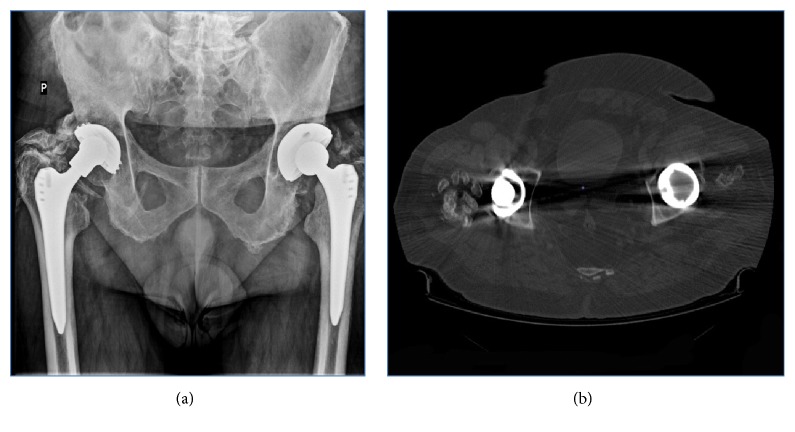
Heterotopic ossification visible on an AP X-ray (a) and on a computed tomography scan (b) around right hip. It is a complete hip ankylosis. HO like this can completely sabotage functional outcomes of THR limiting the range of motion in all planes. This is why using right prophylaxis is so crucial (from the Department of Orthopaedics and Traumatology of the Musculoskeletal System, Infant Jesus Teaching Hospital, Medical University of Warsaw records).
